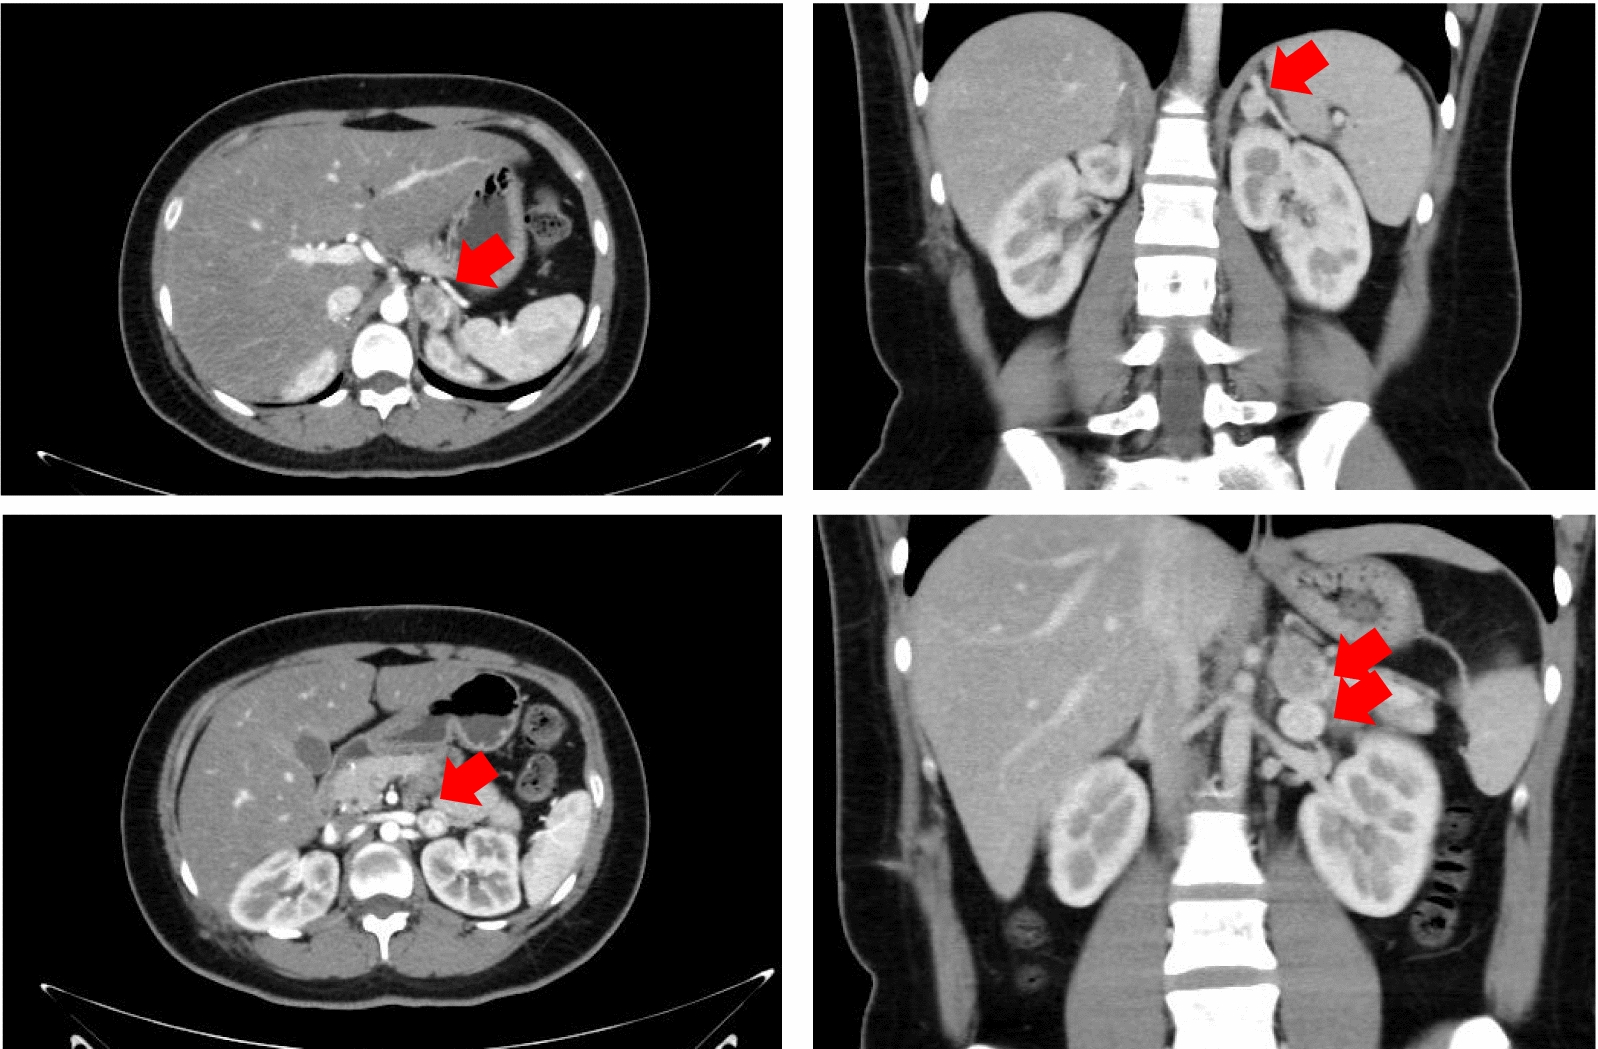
Transverse section and coronal section of CT scans indicated multiple pheochromocytoma and paraganglioma (PPGL) located in left side, which were labelled as red arrow. CT scans indicated that tumor enhancement and central necrosis were obvious
